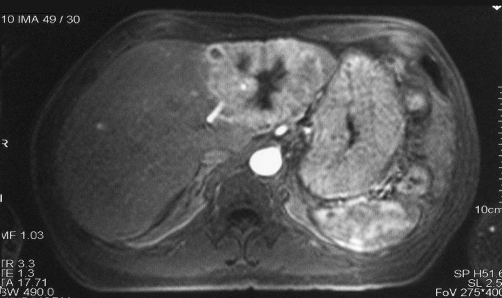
Axial section of MRI showing a left lobe liver mass with central necrosis and contrast uptake.
